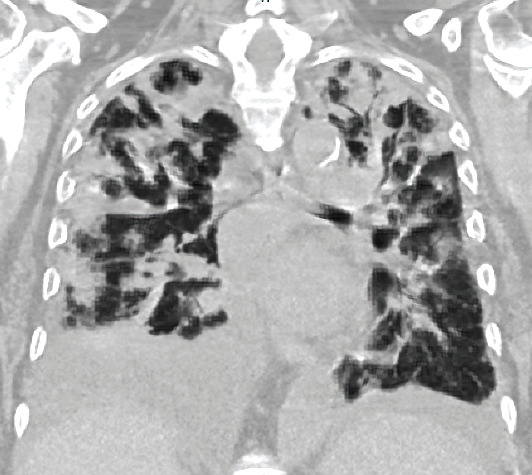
Chest CT scan at presentation to ED post-op concerning for ARDS.
